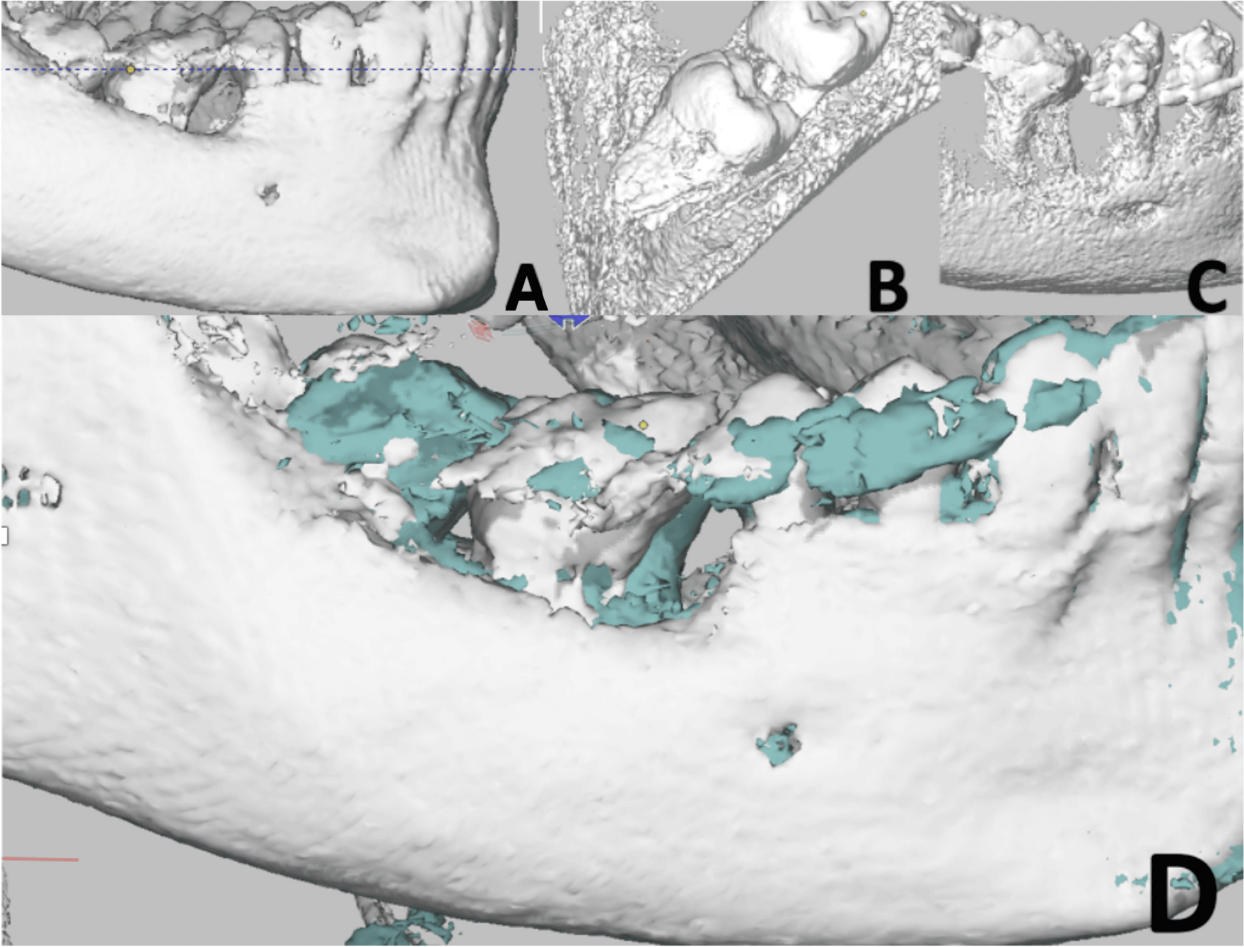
CBCT superimposition Figure 6 shows (A) the mandibular first molar presenting with a bony lesion, (B) the donor tooth in its original position, (C) the donor tooth in its final transplanted position, and (D) the superimposition of the initial CBCT scan with the one-year follow-up scan, aligned on the mandibular incisors, where white indicates the pre-operative image and green represents the post-ATT image. ATT, autogenous tooth autotransplantation; CBCT, cone-beam computed tomography.
